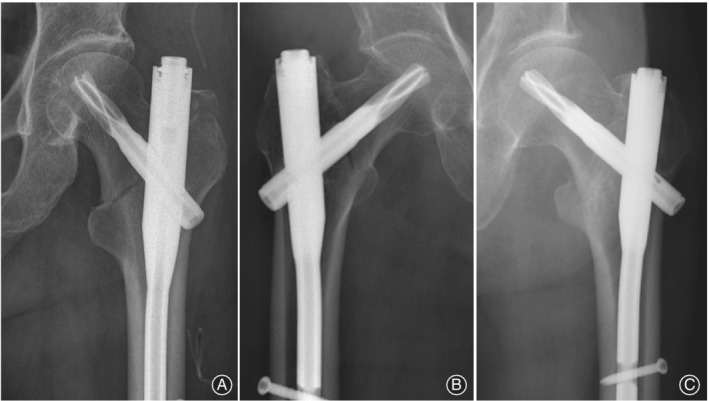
Postoperative radiograph of a typical basicervical intertrochanteric fracture: (A) Shanghai Punan Hospital, (B) Shanghai General Hospital, and (C) Shanghai Seventh People's Hospital.
